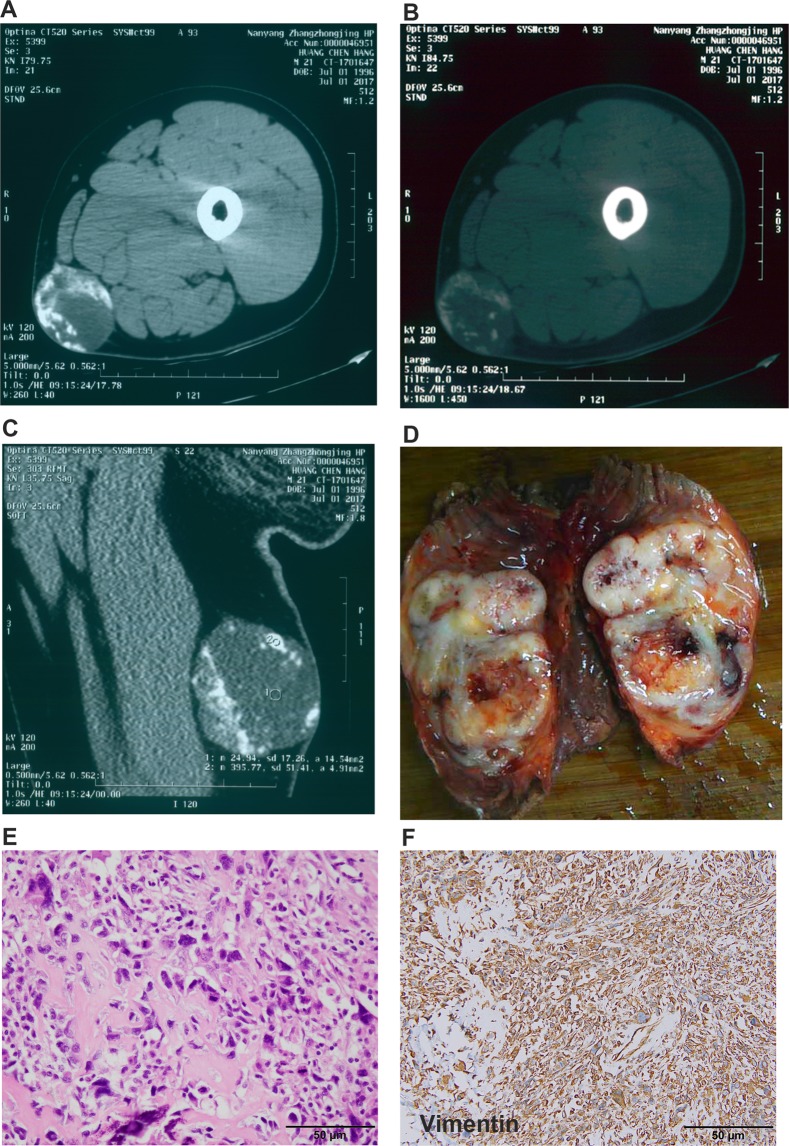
Typical leg ESOS. (A–C) CT scan showed big mass with calcification. (D–F) The pathological diagnosis was confirmed the ESOS.
